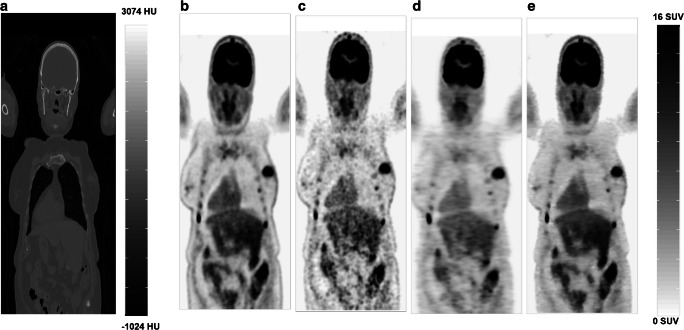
Representative ^18^F-FDG WB PET image of a 66-year old female patient. a Low-dose CT images used for attenuation correction, b reference FD images, c the corresponding LD images, and the predicted FD images using d ResNet (RNET) and e CycleGAN (CGAN)
